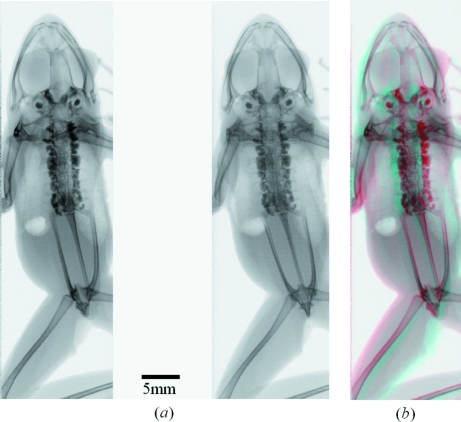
(*a*) X-ray stereo image of the whole body of a frog. This image was digitally combined from sequential images which were acquired by translating the sample vertically. This can be viewed in three dimensions by stereopsis. (*b*) Anaglyph created from the X-ray stereo image.
